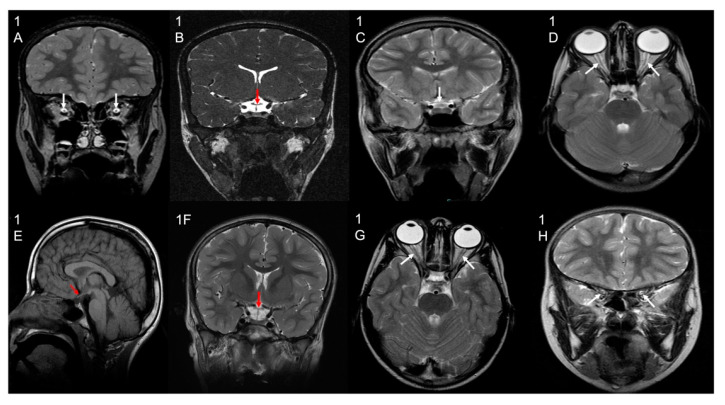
Brain MRI results. Selected MRI sequences and planes showing optic chiasm atrophy (white/red/black arrows) in patient 1 (image (1B) coronal CISS), Patient 2 (image (1C) coronal T2), Patient 4 (image (1E) sagittal T1), and Patient 5 (image (1F) coronal T2). Other selected images also show bilateral optic nerve atrophy (white arrows) in patient 1 (image (1A) coronal T2), Patient 3 (image (1D) axial T2), and Patient 6 (image (1G) axial T2; image (1H) coronal T2).
